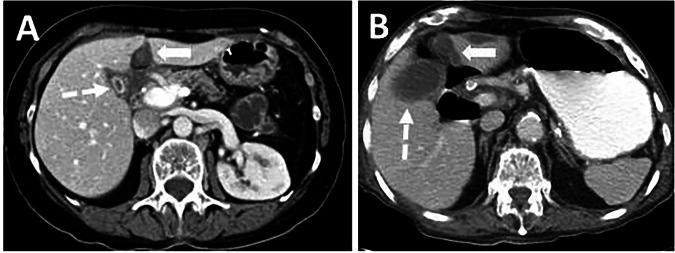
Two different patients with acute cholecystitis. A Axial plane CT image shows the wall thickening of the gallbladder and mucosal enhancement (dashed arrow). Inflammation is also observed in the FL and LTH (arrow). B Axial plane CT image shows the mildly distended gallbladder with wall thickening (dashed arrow). A fluid collection in the FL is also noted (arrow)
